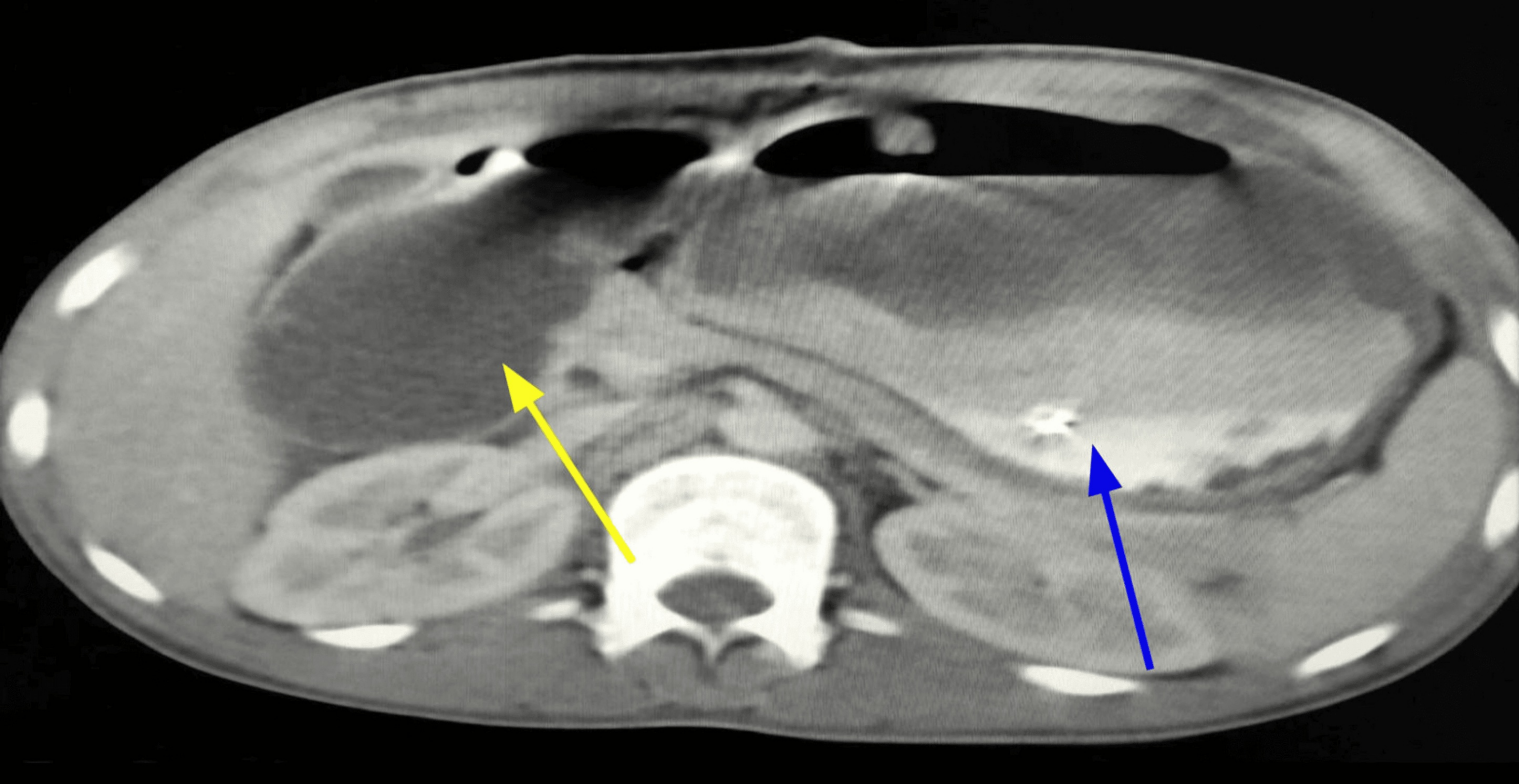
A contrast-enhanced computed tomography (CECT) of the abdomen showing a dilated stomach (blue arrow) and a dilated third segment of the duodenum (yellow arrow)
